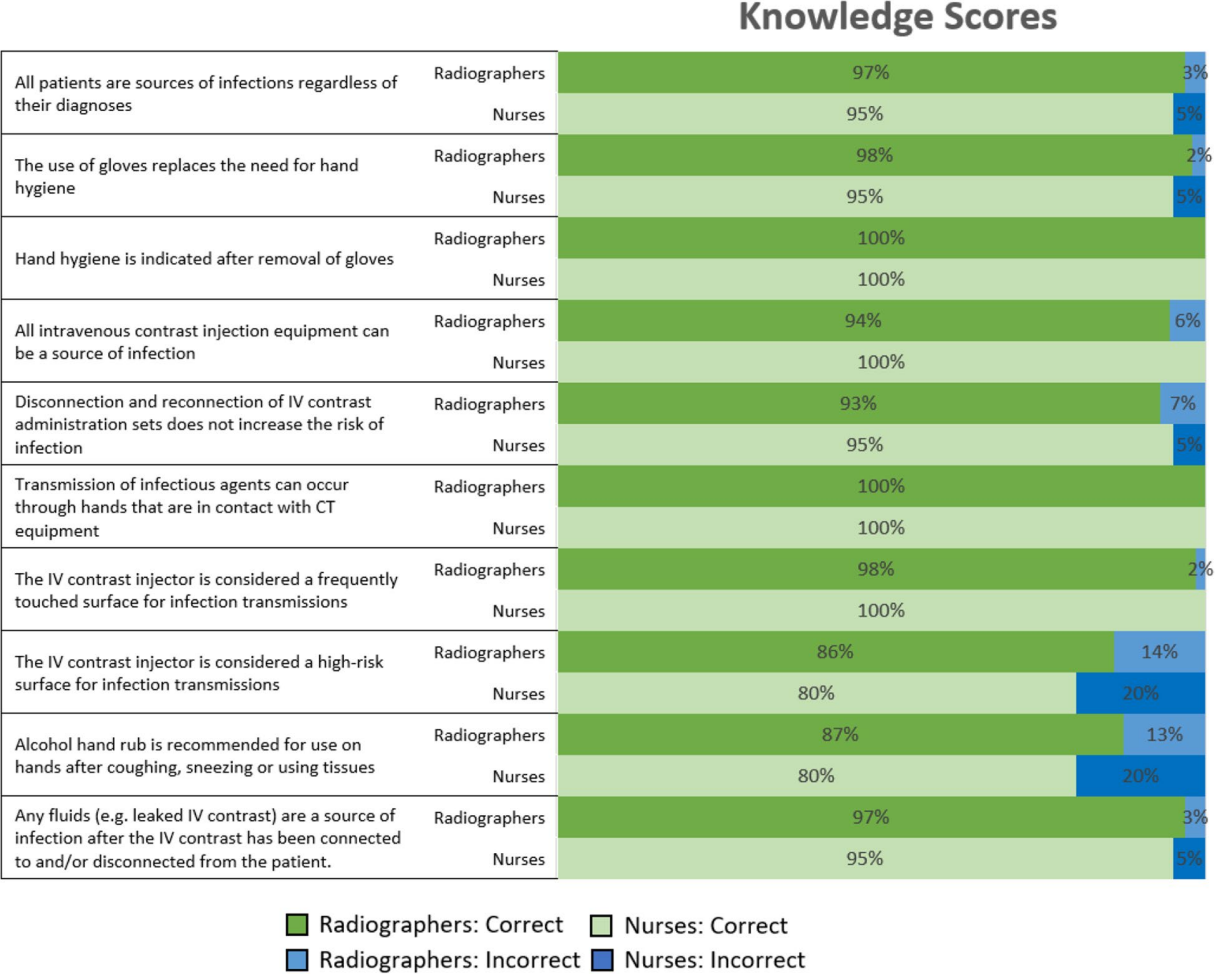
Knowledge questions and responses for radiographers (*n* = 127) and nurses (*n* = 20)
